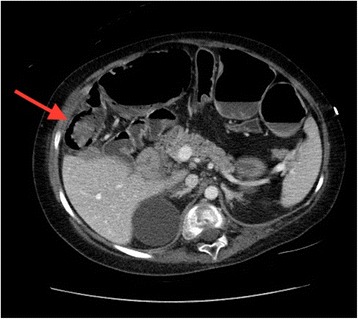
Pneumatosis of the right colon and significant colonic distention identified on computed tomography scan. The arrow is pointing to pneumatosis of the right colon
